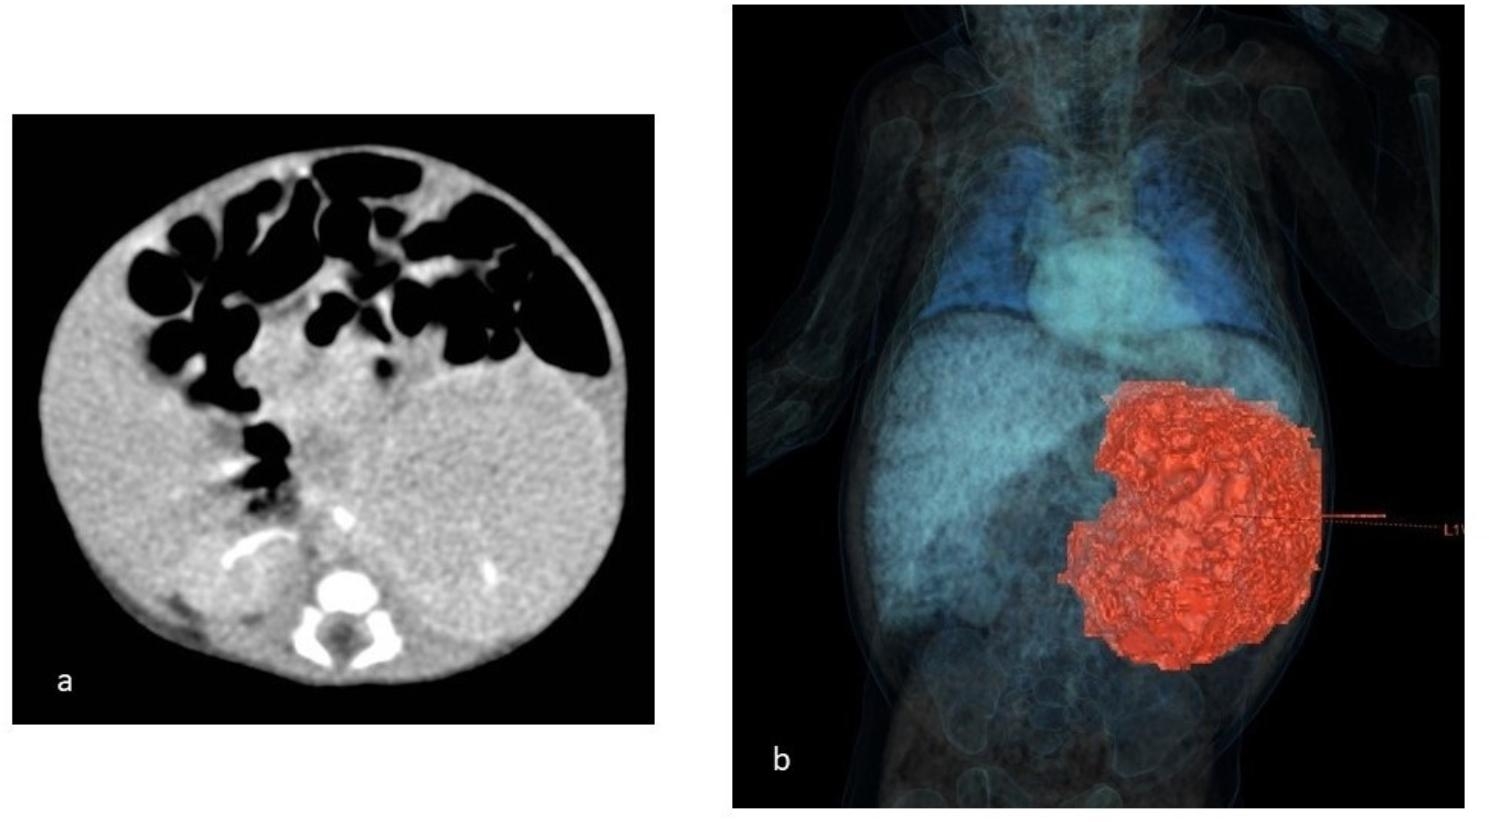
Patient 1. a Abdominal computed tomography shows the left renal mass (corresponding to a volume of 76 mL), and defines its relationships with the surrounding organs and structures. b Three-dimensional CT reconstruction
